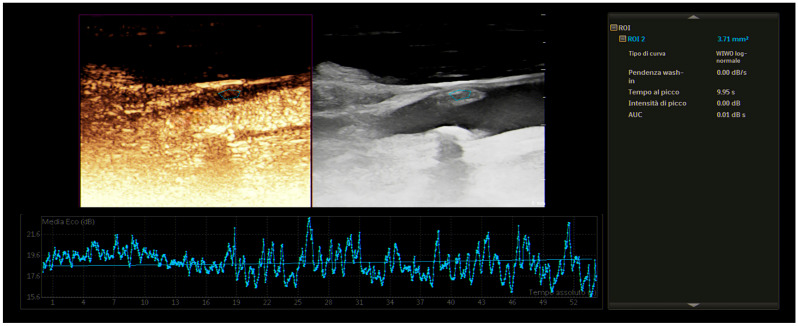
Negative perfusion result on bolus-based wash-in/wash-out log-normal analysis*. Negative perfusion result on bolus-based wash-in/wash-out log-normal analysis. TIC analysis of an internal carotid plaque with curvilinear blue ROI placement on the grayscale B-mode image. The oscillating blue trace represents the raw frame-by-frame contrast-enhanced ultrasound signal, which remains close to background noise. The continuous blue line represents the fitted log-normal model and shows no meaningful contrast-related increase, supporting absence of measurable intraplaque enhancement. This pattern should be distinguished from technically inadequate acquisitions.
